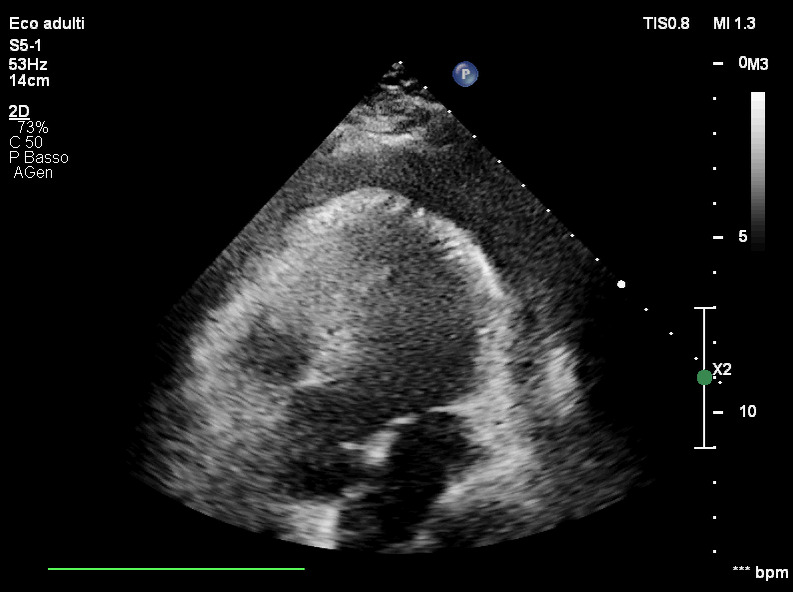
Transthoracic echocardiography (subcostal five-chamber view) showing a circumferential pericardial effusion. Representative subcostal TTE image demonstrating an anechoic pericardial fluid layer surrounding the heart, consistent with circumferential pericardial effusion. This first-line view is commonly used in emergency and ICU settings to rapidly confirm effusion presence and guide escalation to hemodynamic assessment and drainage when tamponade physiology is suspected. ICU, intensive care unit; TTE, transthoracic echocardiography; MI, mechanical index; 2D, two-dimensional echocardiography; bpm, beats per minute.
